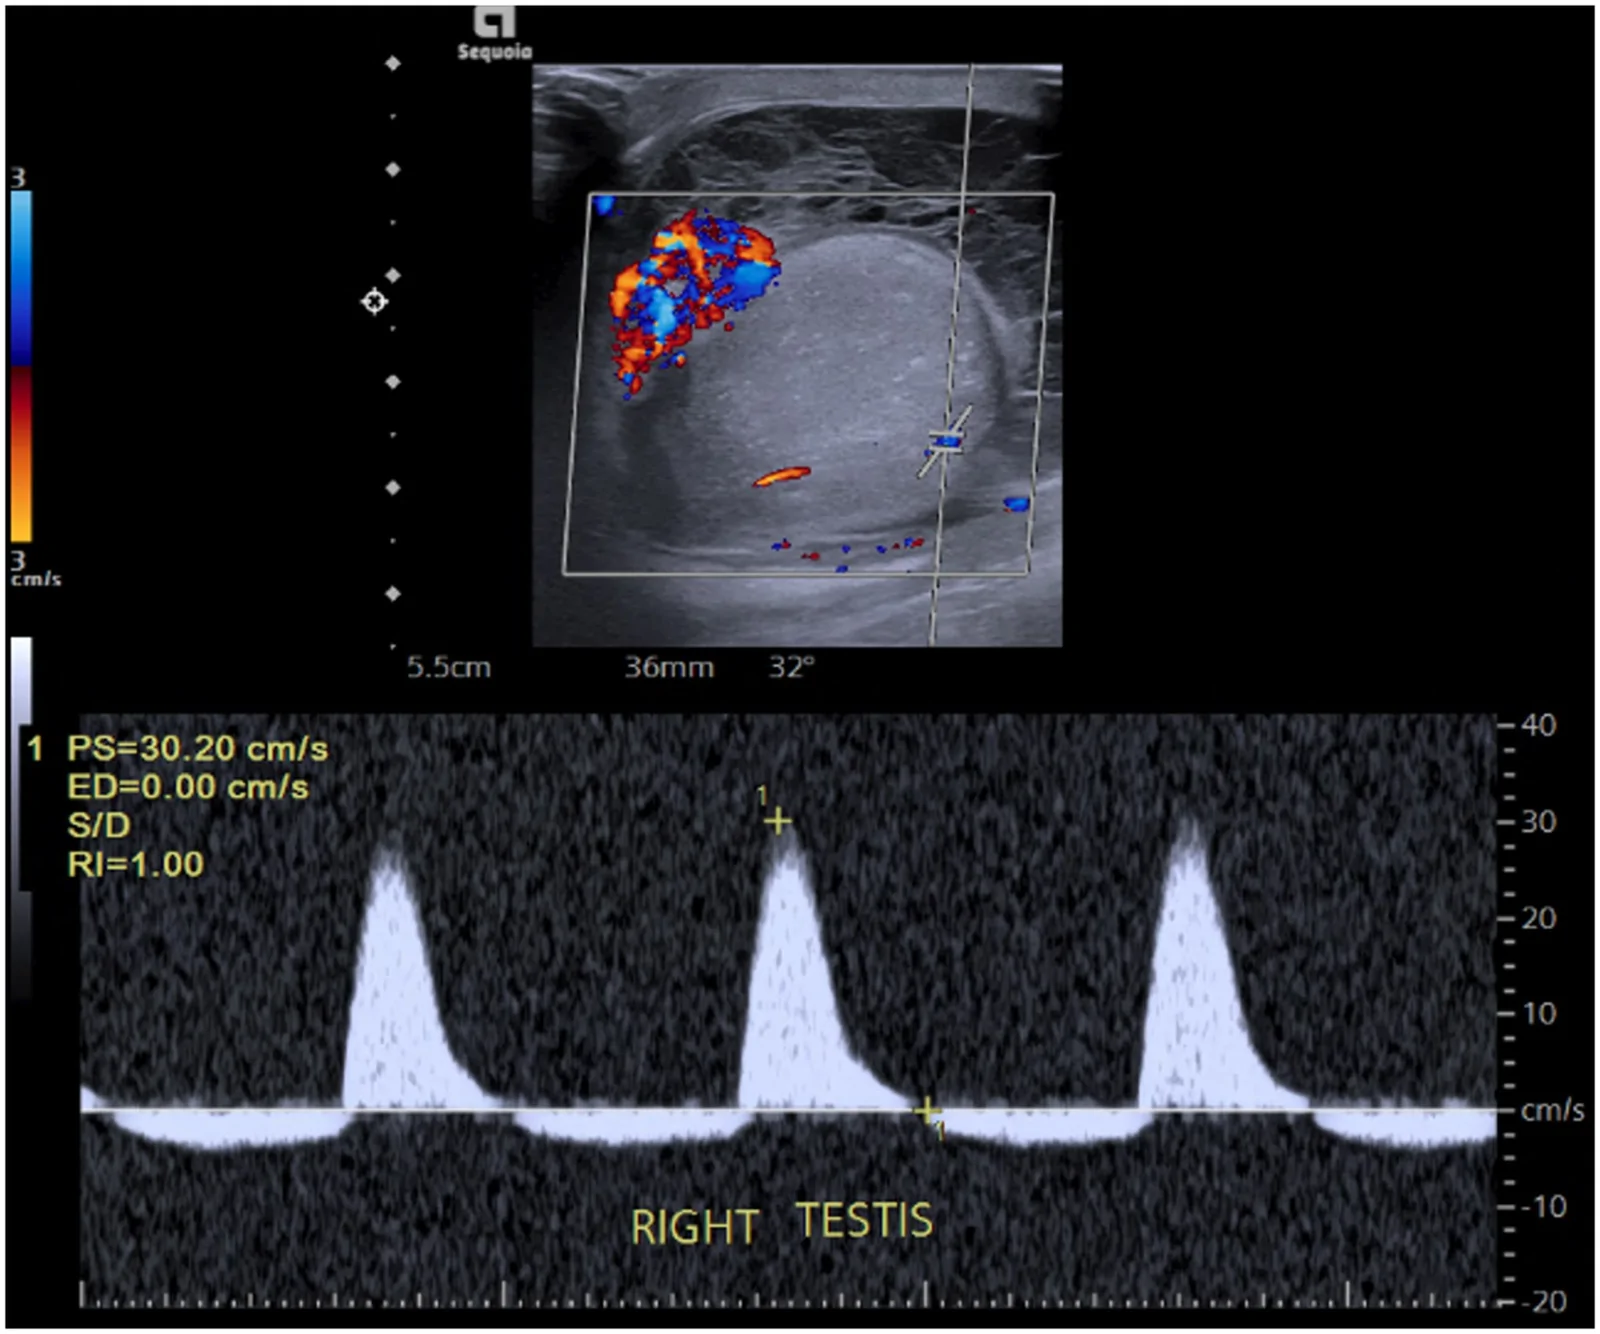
Repeat spectral Doppler ultrasound of the right testis on day 8. Pulsed-wave spectral Doppler of residual intratesticular arterial flow was obtained using a Siemens ACUSON Sequoia ultrasound machine with a 15L4 linear transducer and testicular preset. Color Doppler scale was ±3 cm/s with PRF 625 Hz. Spectral Doppler was obtained with a gate size of 1.5, PRF 5435 Hz, filter setting 60, and Doppler angle 32°, demonstrating persistent high-resistance arterial flow with reversed diastolic flow; PSV 30.20 cm/s, EDV 0.00 cm/s, and RI 1.00.
